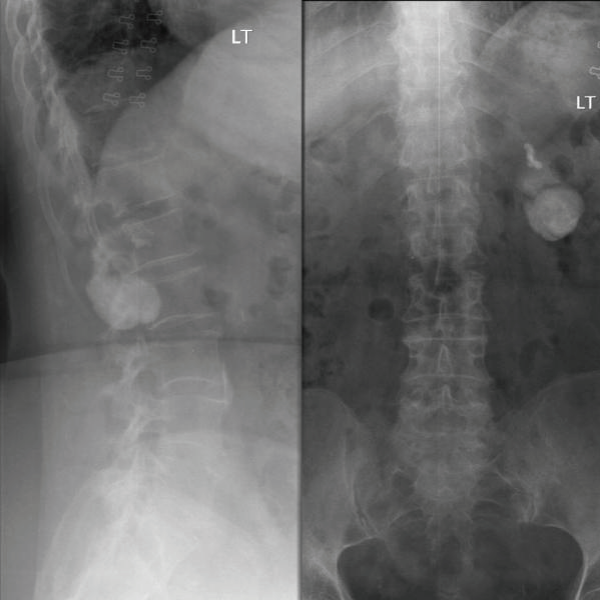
AP & Lateral lumbar spine X-rays.
